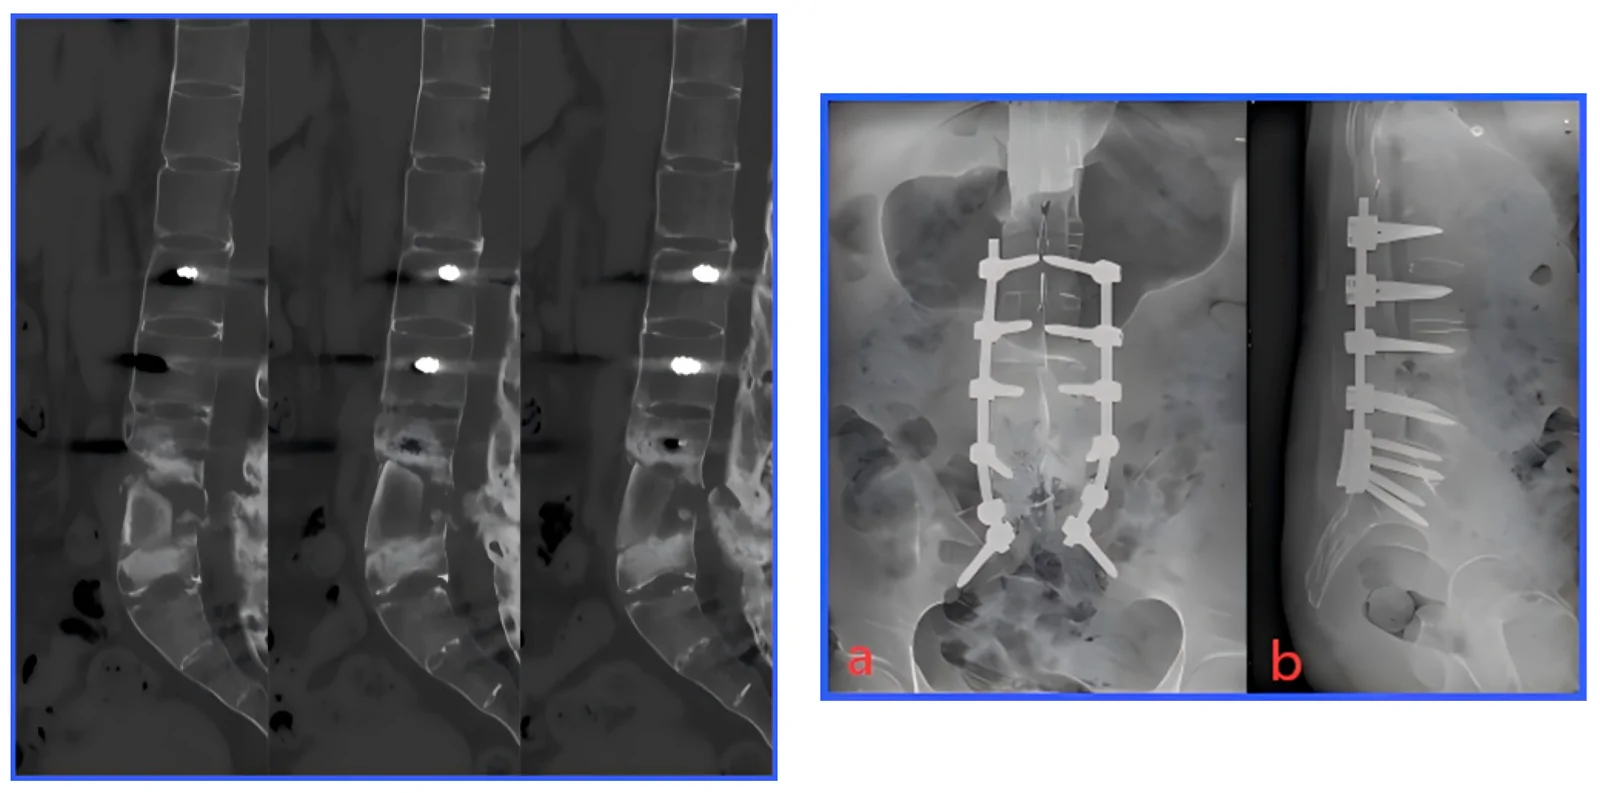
Early postoperative imaging. (a) Anteroposterior and lateral radiographs show maintained alignment and satisfactory long-segment instrumentation. (b) Computed tomography confirms appropriate position of the posterior fixation construct and anterior structural graft.
